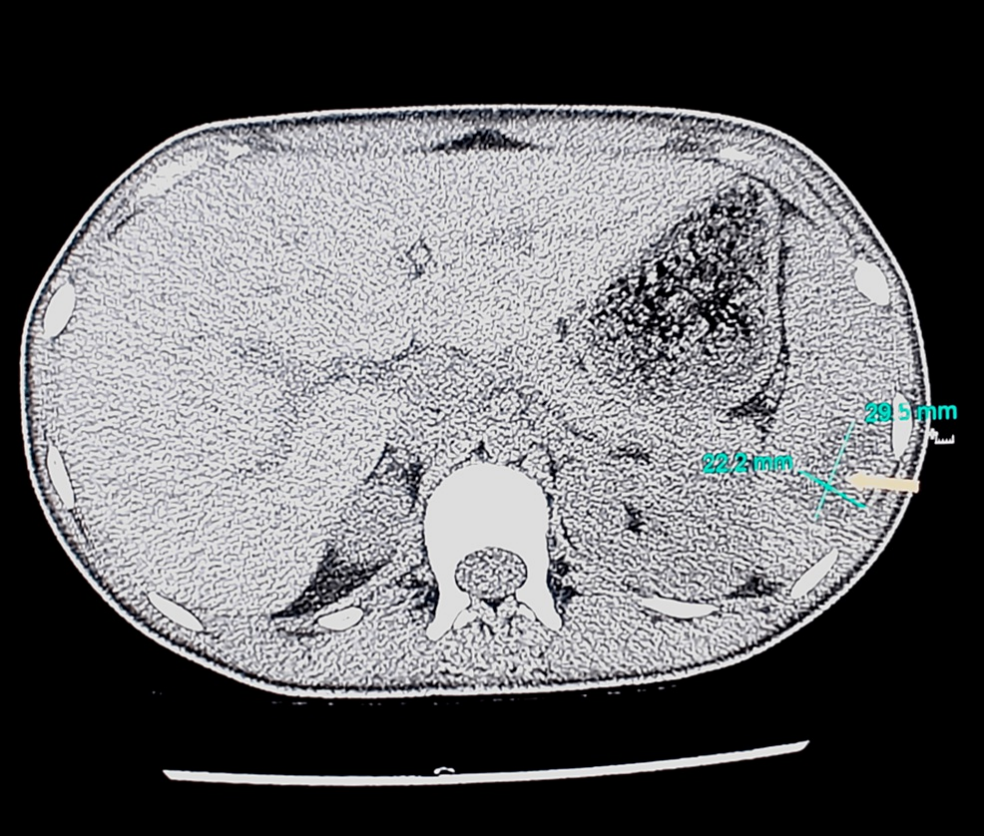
CT abdomen showing abscess in inferior pole of spleen of size 2.9 cm x 2.2 cm (arrow)
